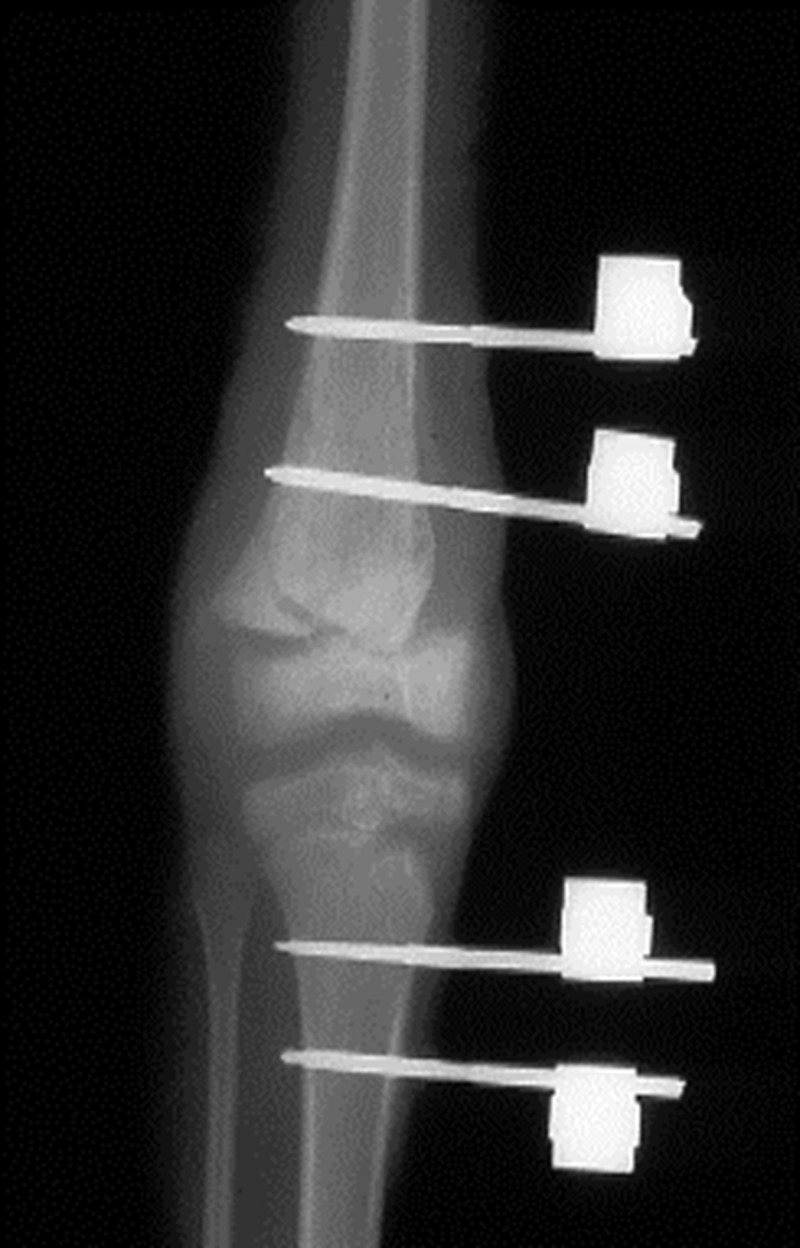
Radiograph showing spanning external fixator in place.
